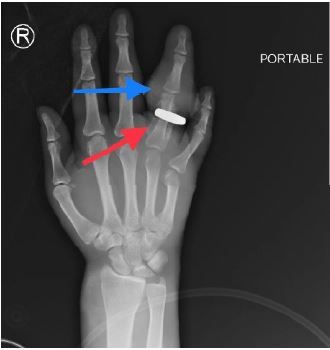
Radiograph of right hand with marked fourth digit swelling distal to the patient’s ring suspicious for tourniquet syndrome. The blue arrow shows soft tissue swelling and the red arrow shows periosteal elevation. Periosteal reaction involving proximal fourth digit phalanges suspicious for osteomyelitis (both arrows).
